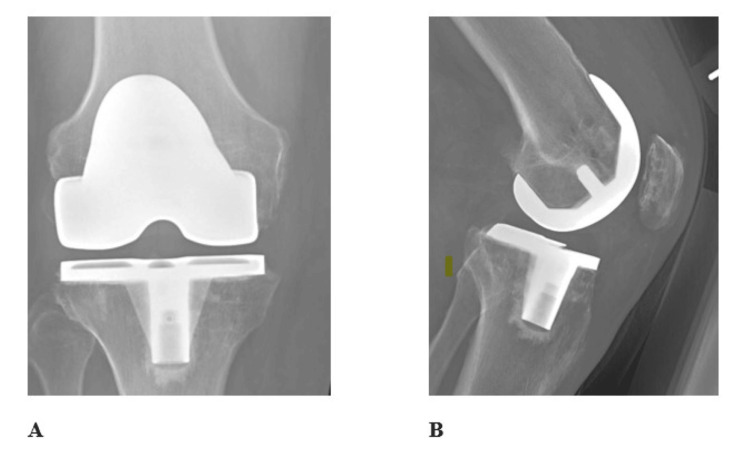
A participant underwent ISA removal after two years of follow-up and conversion to TKA at four years Five-year anteroposterior (A) and lateral (B) radiographs are presented above. TKA was performed as a primary arthroplasty procedure with no additional hardware and no evidence of implantable shock absorber-related complications. ISA: implantable shock absorber; TKA: total knee arthroplasty
